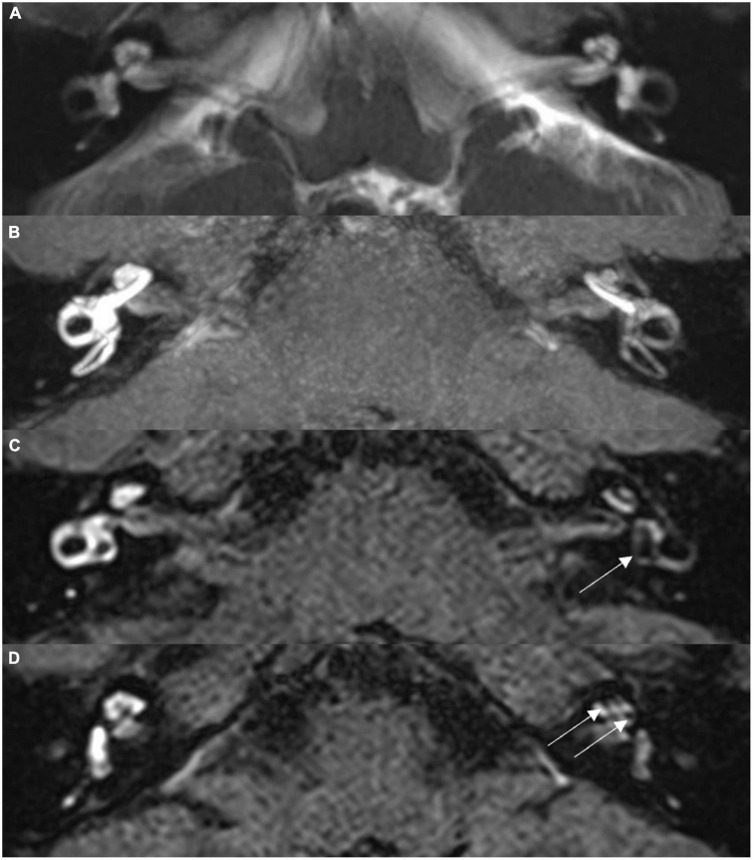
Magnetic resonance imaging (MRI) scans for a 36-year-old male with left-sided Ménière’s disease. (A) Routine MRI hydrography image, maximum intensity projection, showing symmetrical signal for bilateral labyrinth structures, without abnormality. (B) Isotropic 3D-SPC inversion recovery FLAIR image, maximum intensity projection reconstruction, showing narrowed left cochlear canal and significantly low signal in the vestibule. (C) Thin section original image from scan (B); arrow indicates dilated sacculus and utriculus. (D) Thin section original image from scan (B); arrow indicates the expanded scala media of the cochlea.
